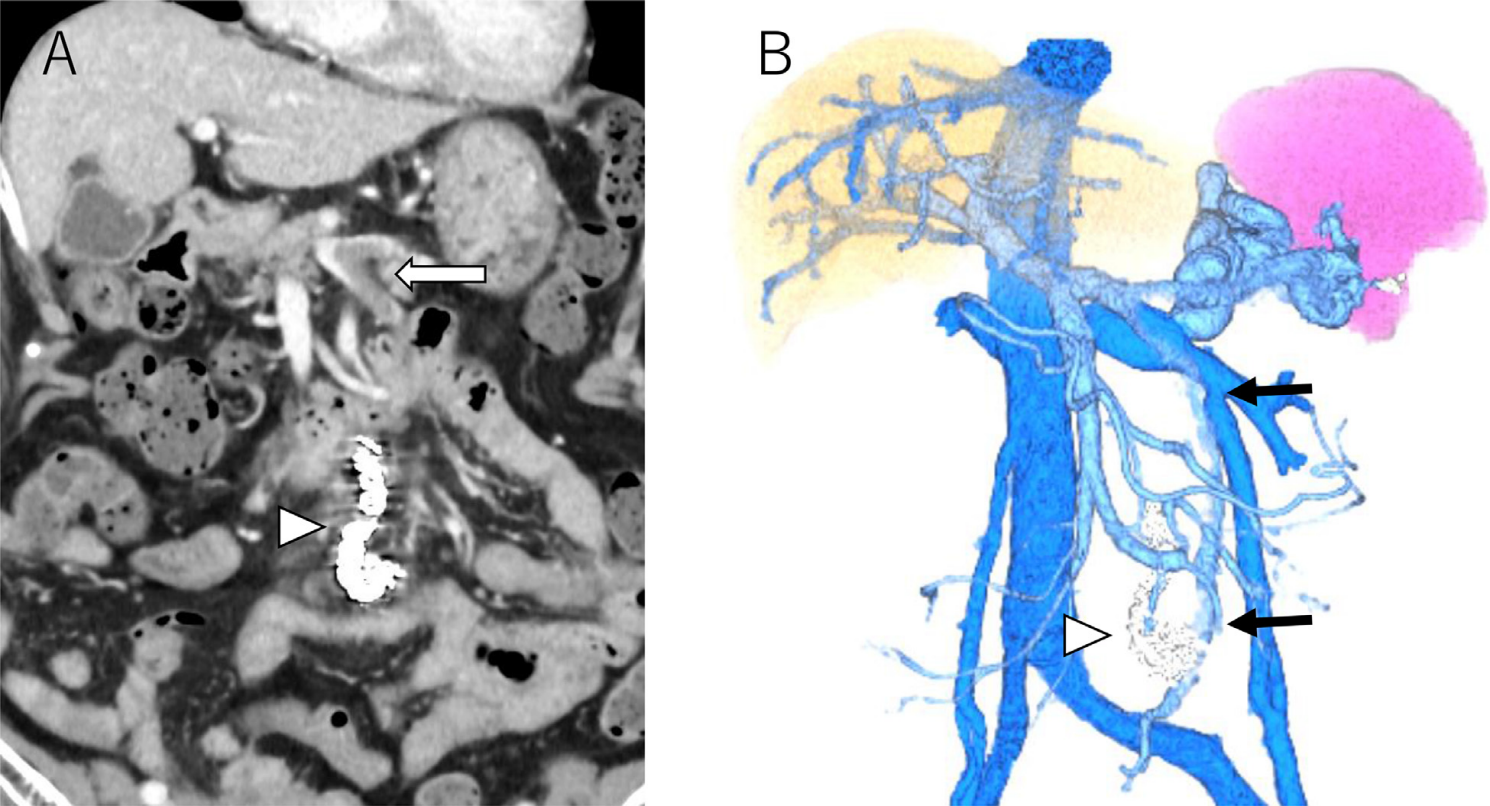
Contrast-enhanced CT and 3D-CT reconstruction images after treatment. (A) Coronal view of Contrast-enhanced CT shows the thrombus (white arrow) in the IMV and the splenic vein. White arrowhead indicates embolized microcoils. (B) 3D-CT reconstruction image after PSE and RTO shows the thrombus (black arrow) in the IMV. White arrowhead indicates embolized microcoils.
